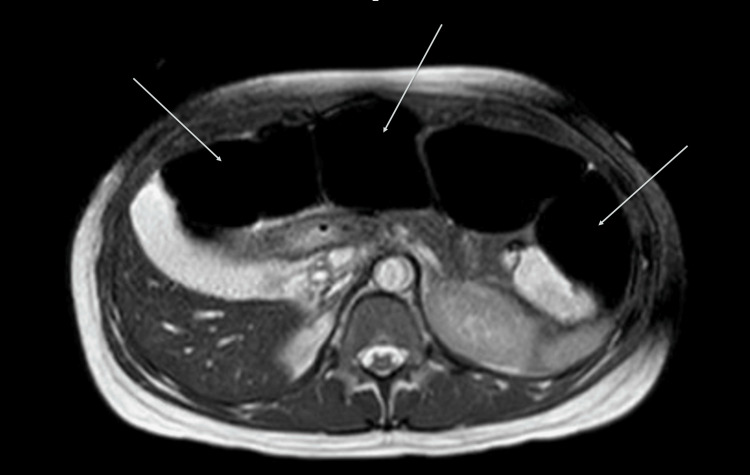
MRI of the abdomen without intravenous (IV) contrast. Moderate to severe dilation of the transverse colon and right colon with a change of caliber at the level of the proximal descending colon.
